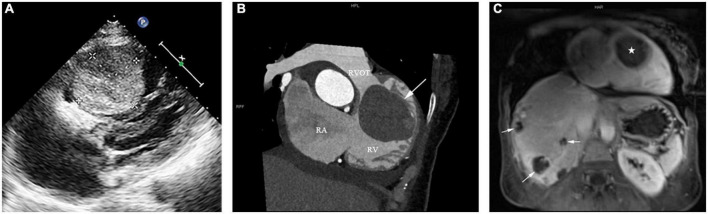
(A) Preoperative transthoracic echocardiography showing a large mass measuring 4.82 cm × 4.48 cm × 6.42 cm attached to the anterior wall of the right ventricle, with a moderate to strong and relatively homogeneous echo. (B) The computed tomography scan of the patient’s chest showing a right ventricular mass (arrow) in the right ventricular outflow tract (RVOT). RA: right atrium; RV: right ventricle. (C) Reconstructed magnetic resonance imaging of the patient’s chest and abdomen showed a cardiac mass concurrent with multiple hepatic lesions. The right ventricular mass and the multiple liver lesions are indicated by a star and three arrows, respectively.
